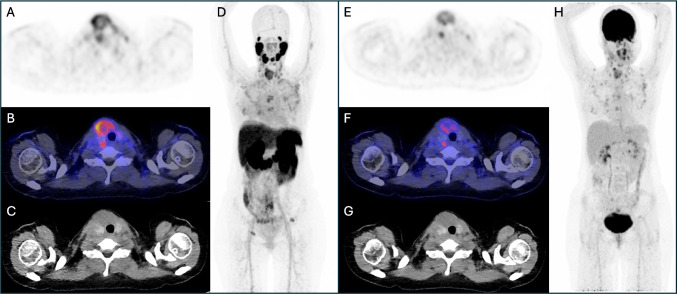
Images of ^18^F-PSMA-1007 PET/CT (left) and ^18^F-FDG PET/CT (right) in a medullary thyroid cancer patient with extensive distant metastatic disease in the thyroid, locoregional lymph nodes, lungs and bone. A-C: transverse view at the level of the primary thyroid tumor on ^18^F-PSMA-1007 PET/CT, D: maximum intensity projection (MIP) of ^18^F-PSMA-1007 PET/CT, E–G: transverse view at the level of the primary thyroid tumor on ^18^F-FDG PET/CT (same patient), H: MIP of ^18^F-FDG PET/CT. The authors affirm that the human research participant provided informed consent for publication of the images in this figure
